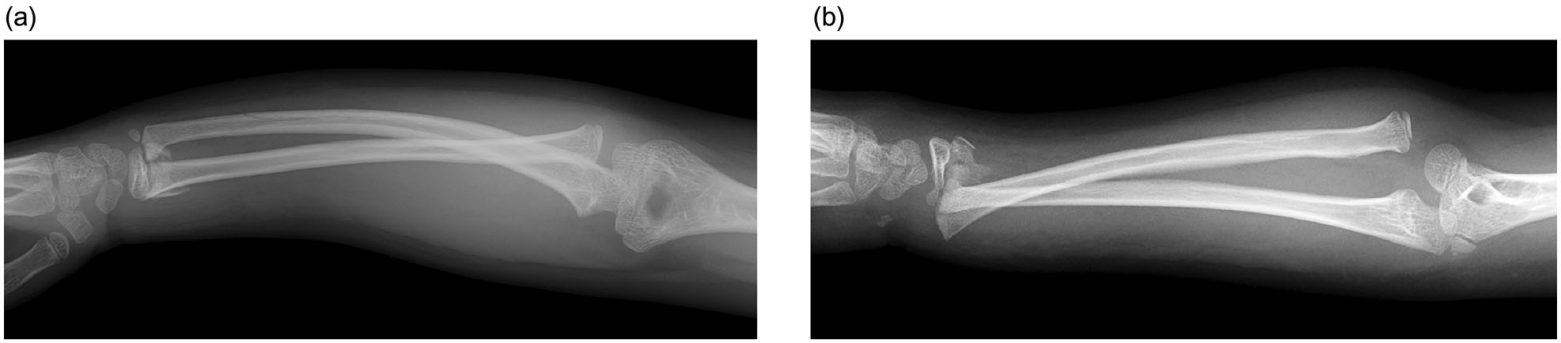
(a, b) Radiographs of the right forearm at the first presentation. Note that true lateral view was not obtained at this point.
